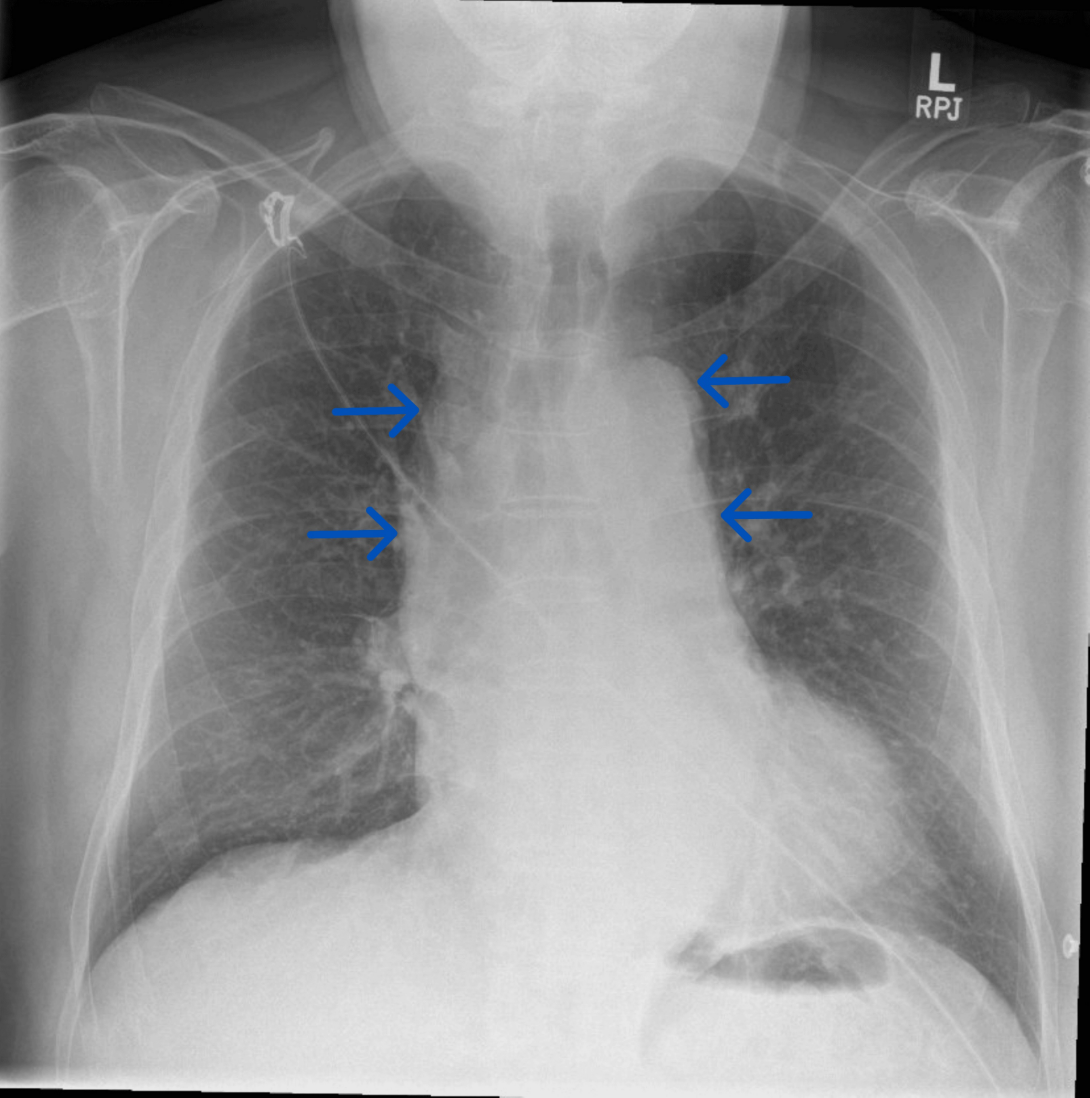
Chest radiograph at hospital admission. A chest radiograph obtained at admission showing a widened mediastinum (blue arrows), initially thought to indicate aortic unfolding from longstanding systemic hypertension.
